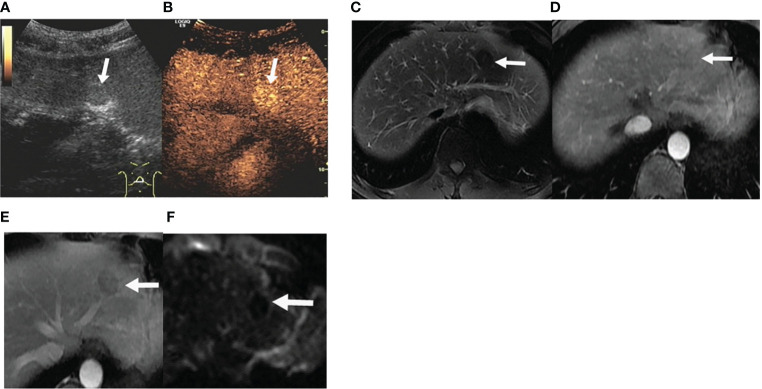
Case 2 in our hospital. (A) US demonstrated a round mass in the segment II of the liver near the dome of the diaphragm, with even slightly hyperecho, measuring about 1.9 cm ×1.6 cm. (B) CEUS was performed and revealed hyperenhancement in the delayed phase (white rows). (C) The mass in the segment II of the liver (white rows) revealed low T2 signal. (D, E) Enhanced MR showed an arterial enhancement and had a gradual washout in the venous phases. (F) On DWI, no restricted diffusion was shown in the mass.
